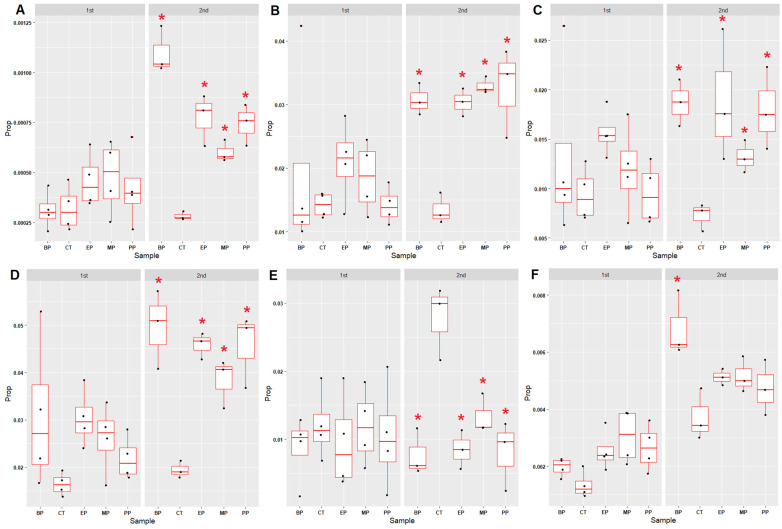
Proportion changes in the nitrogen cycle-associated microbial communities in the paraben-treated river sediments. (A) Anammox (anaerobic ammonium oxidation) (B) Nitrogen fixation (M00175: nitrogen => ammonia) (C) Denitrification (M00529: nitrate => nitrogen) (D) Dissimilatory nitrate reduction (M00530: nitrate => ammonia) (E) Nitrification (M00528: ammonia => nitrite) (F) Assimilatory nitrate reduction (M00531: nitrate => ammonia). “1st” indicates the meaning of the period between week 0 and week 8. “2nd” indicates the meaning of the period between week 8 and week 17. Red star indicates the *p* value of the Mann–Whitney U test < 0.05 (compared with control (CT)). Prop: proportions of microbial genera. “M00xxx” indicates the meaning of the KEGG module ID number. MP: methylparaben, EP: ethylparaben, PP: propylparaben, BP: butylparaben, CT: control.
